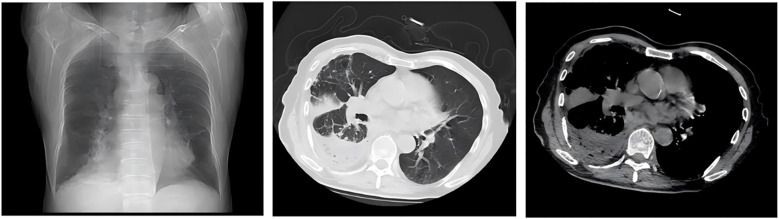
By post-procedure day 7, a repeat chest CT revealed a substantial decrease in both fluid and gas accumulation compared to prior imaging.
